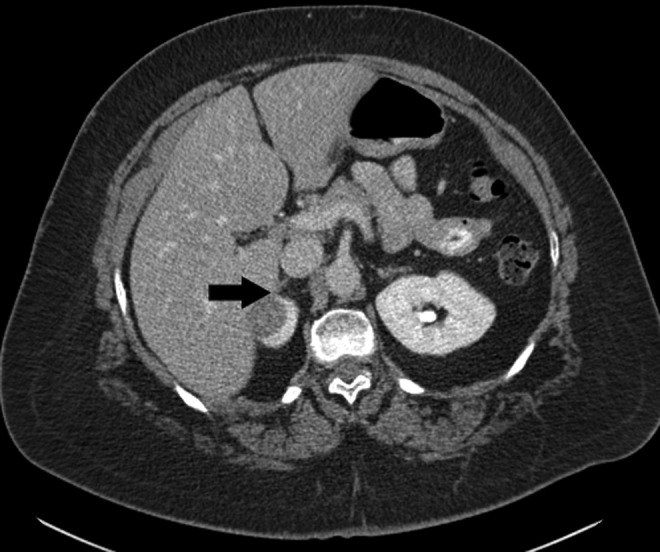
CT image. Transverse cut in cortical phase. An enhancing renal mass (*black arrow*) is seen at the upper pole of the right kidney and is abutting the lateral limb of the right adrenal gland.
